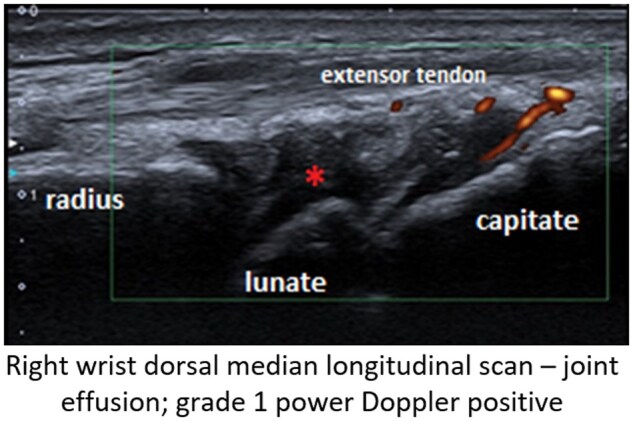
Example image to be included in the ultrasound logbook. The type of scan, important anatomical landmarks and the abnormality should be labelled
